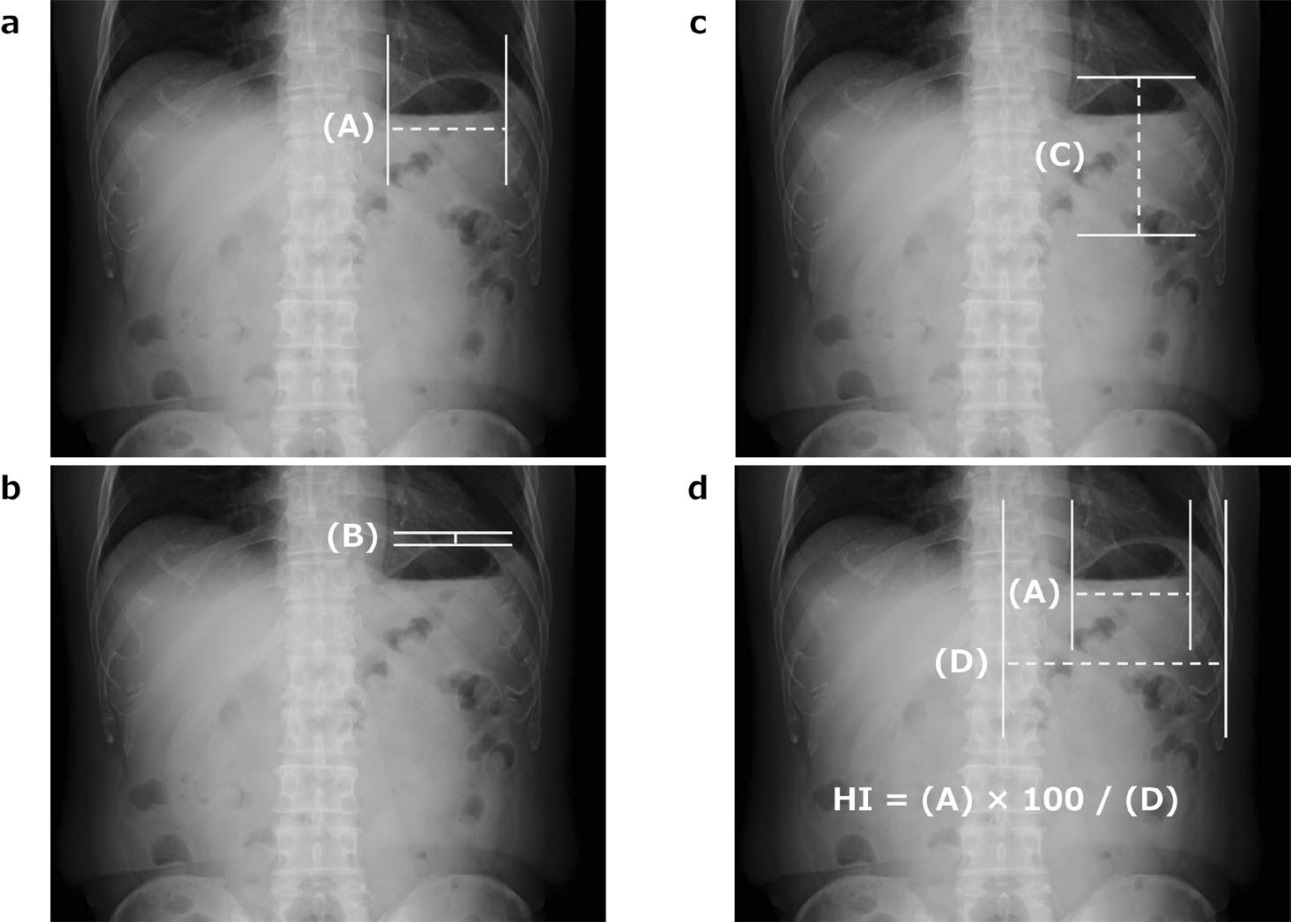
Radiographic measurements used to assess postoperative gastric distension. (a) Maximum horizontal width of the gastric bubble. (b) Vertical distance from the apex of the gastric bubble to the left diaphragm. (c) Head‐to‐tail (longitudinal) length of the remnant stomach. (d) Reference distance from the center of the vertebral body to the left costophrenic angle. The Hiki Index (HI) was calculated as the ratio of each measurement (a) to (d), expressed as a percentage.
